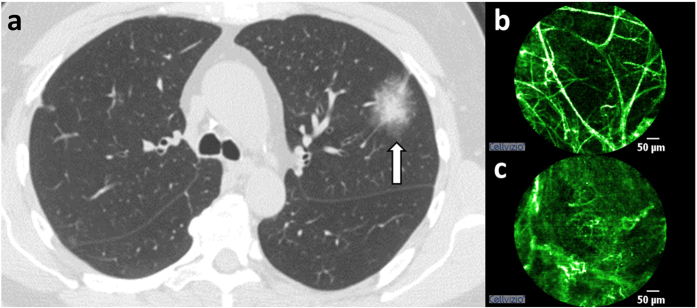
(a) CT scan demonstrating pulmonary nodule, (b) normal elastin structure, (c) abnormal elastin structure at nodule. Notice that abnormal structure may appear due to benign or malignant cause.
